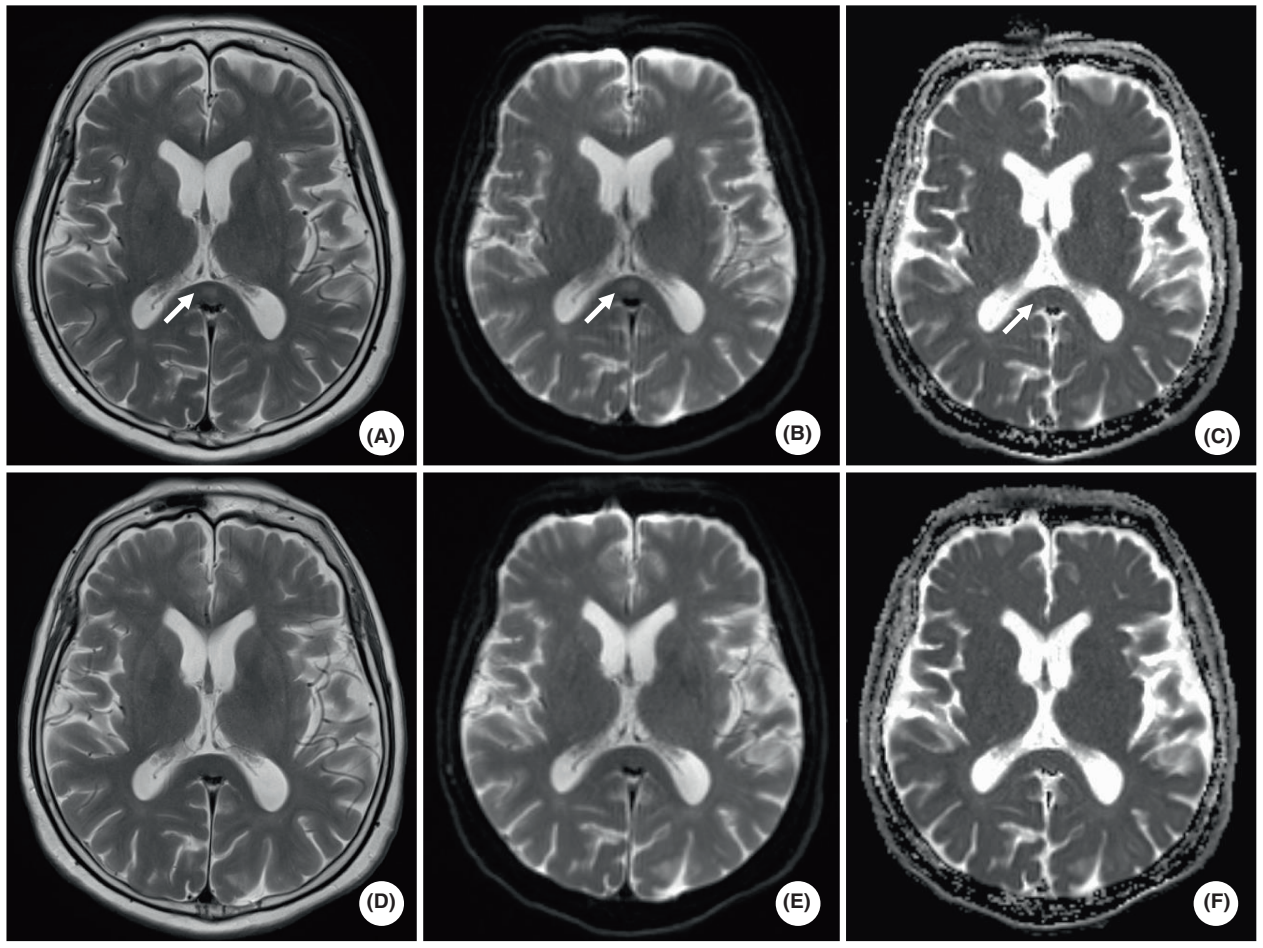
Brain MRI of this case. T2WI (A) and diffusion‐weighted imaging (DWI) (B) reveal hyperintense lesions in the splenium of the corpus callosum. DWI shows low apparent diffusion coefficient values (C). However, the brain MRI 15 days later revealed that the abnormal signal had disappeared in each image (D–F).
